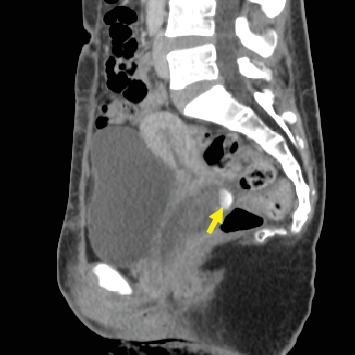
Pelvic computed tomography scan (sagittal view) reveals a large mass, which shows a dappled contrast filling on the arterial phase, located in the posterior vaginal wall (arrow).
